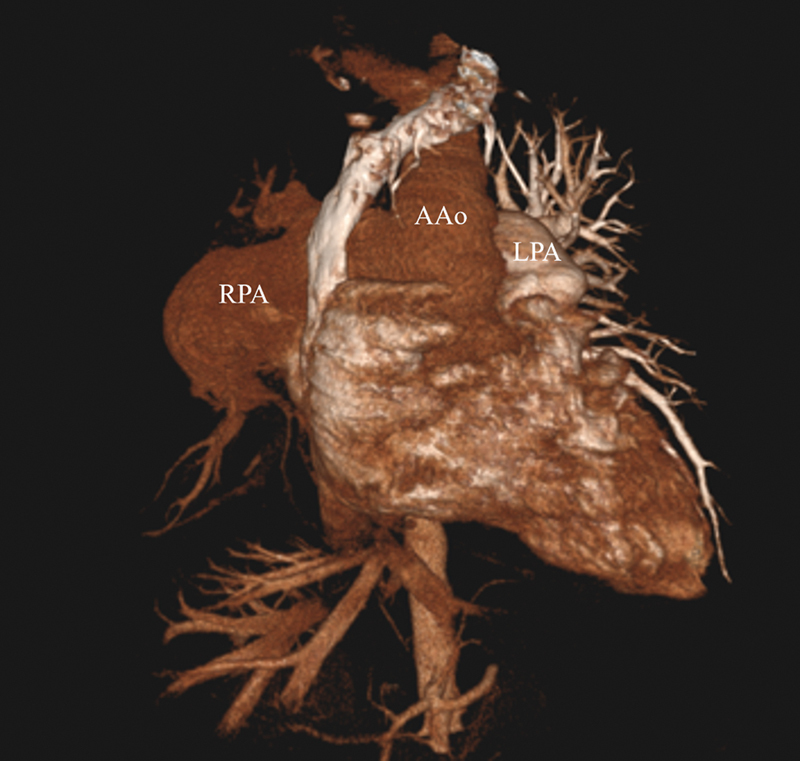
Cinematic rendering from computed tomography angiography (CTA) shows the abnormal right pulmonary artery (RPA) arising from the right lateral wall of the ascending aorta (AAo).
